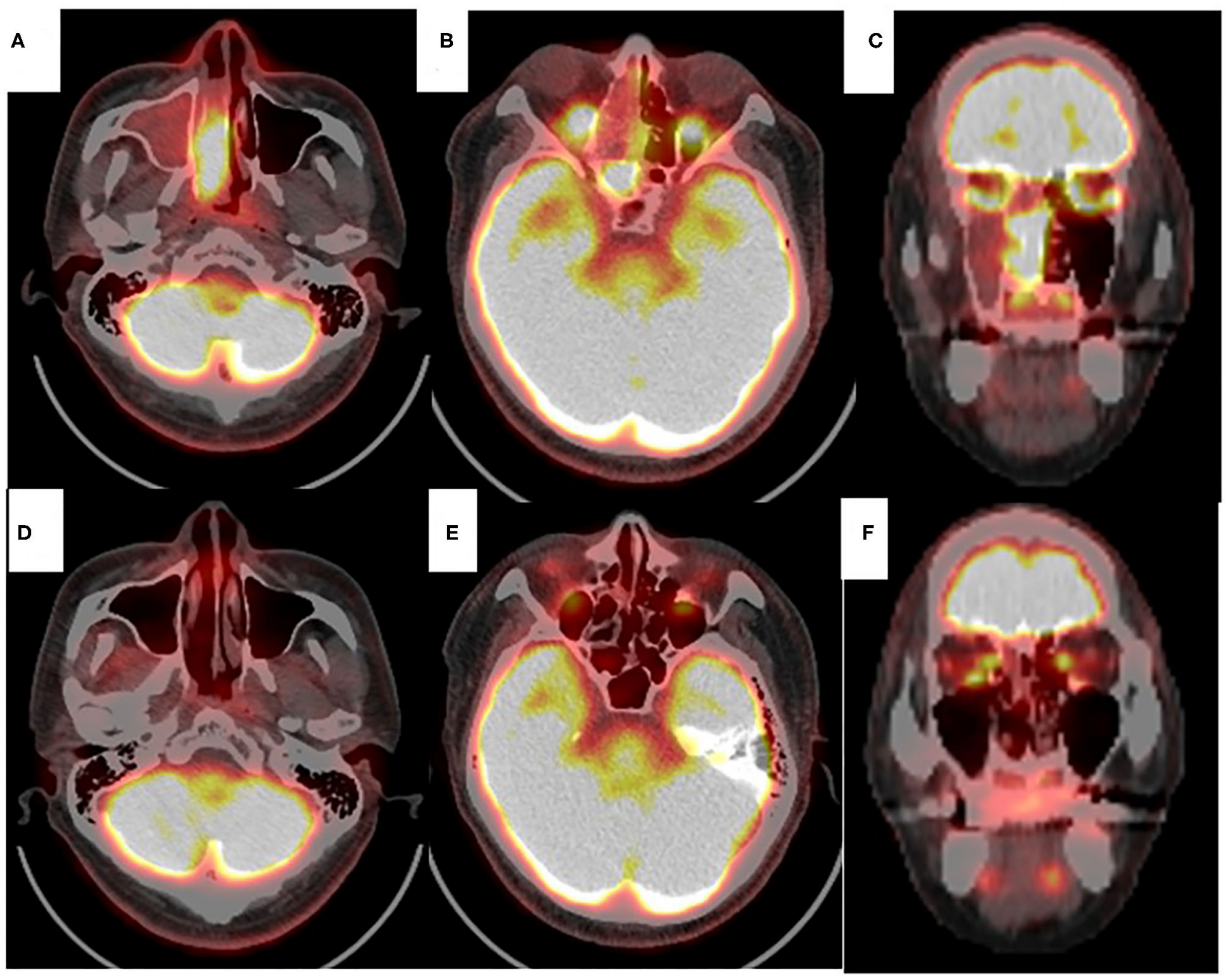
Positron emission tomography with 18F-fluorodeoxyglocose (FDG PET) of the sinonasal region. Axial view (A,B,D,E). Coronal view (C,F). Before chemoradiotherapy (A–C). Significant FDG uptake was found in right nasal cavity, ethmoid and sphenoid sinus. PET scans obtained after chemoradiotherapy (D–F). No significant FDG uptake was found in right nasal cavity or paranasal sinus (D–F).
